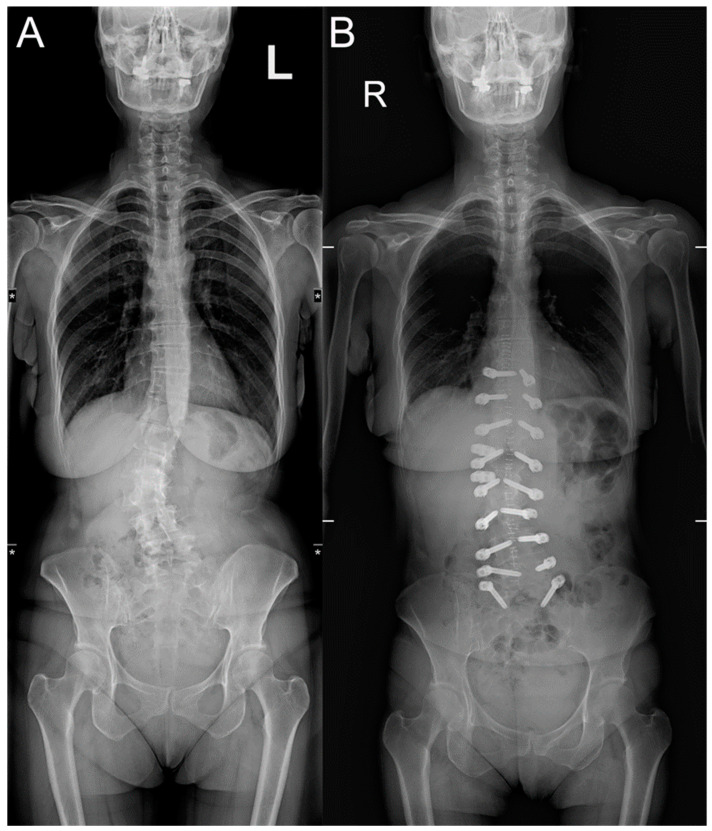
Adult scoliotic deformity treated with Dynesys dynamic stabilization. (A) Pre-operative and (B) post-operative dynamic coronal X-rays.
